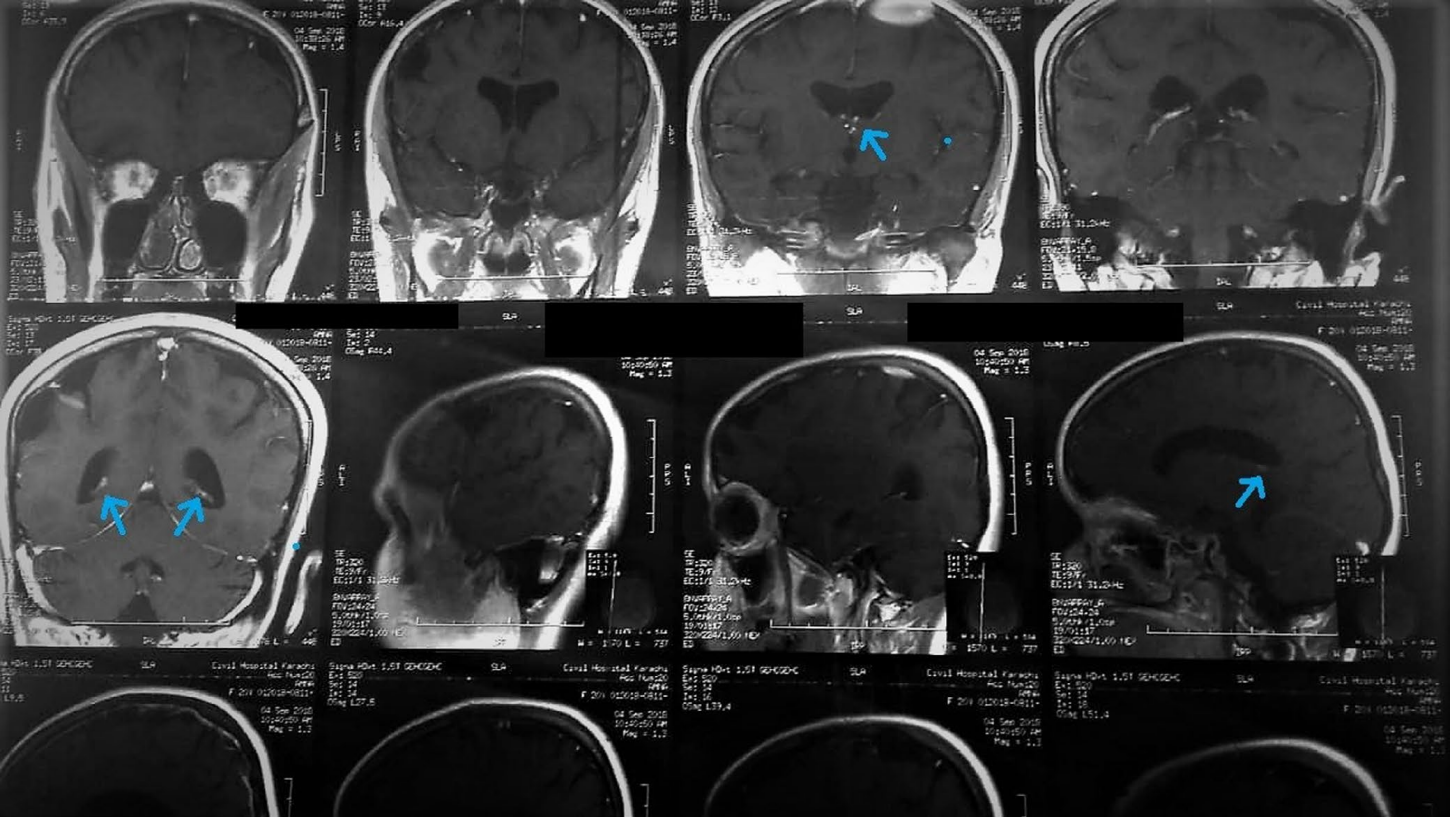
MRI of the brain (sagittal and coronal) shows subependymal nodules (blue) are seen returning isohypointense signals onT2 and hyperintense on T1 without showing post‐contrast enhancement
